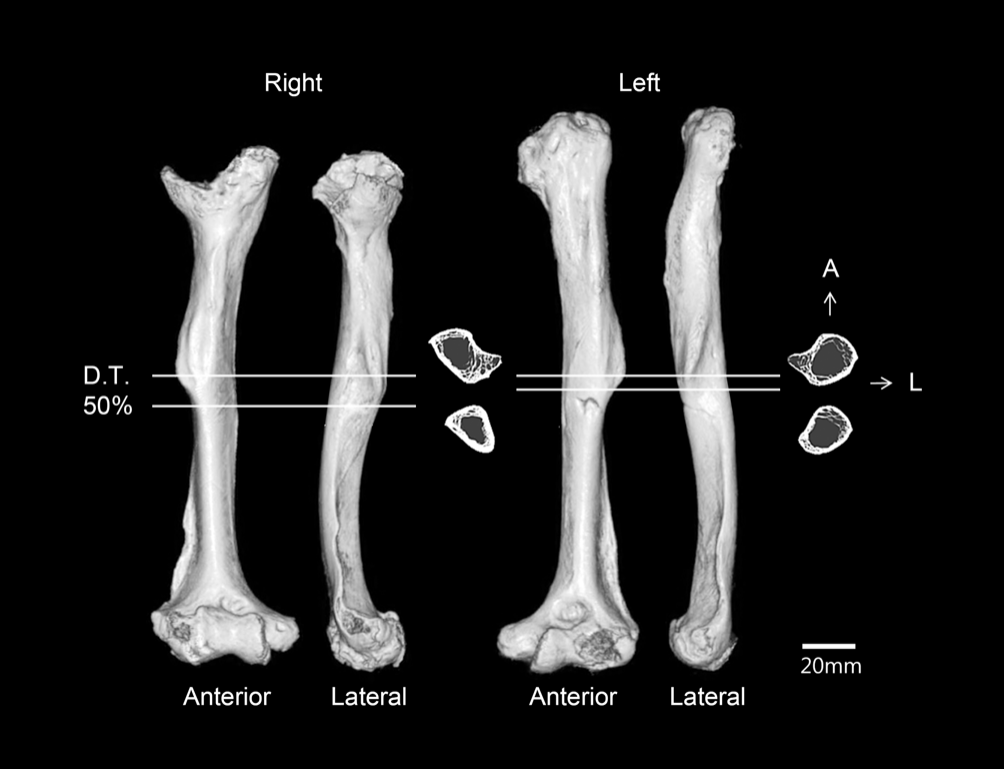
Reconstructed CT images and cross-sectional images of the bilateral humeri (D.T., deltoid tuberosity; A, anterior; L, lateral).
